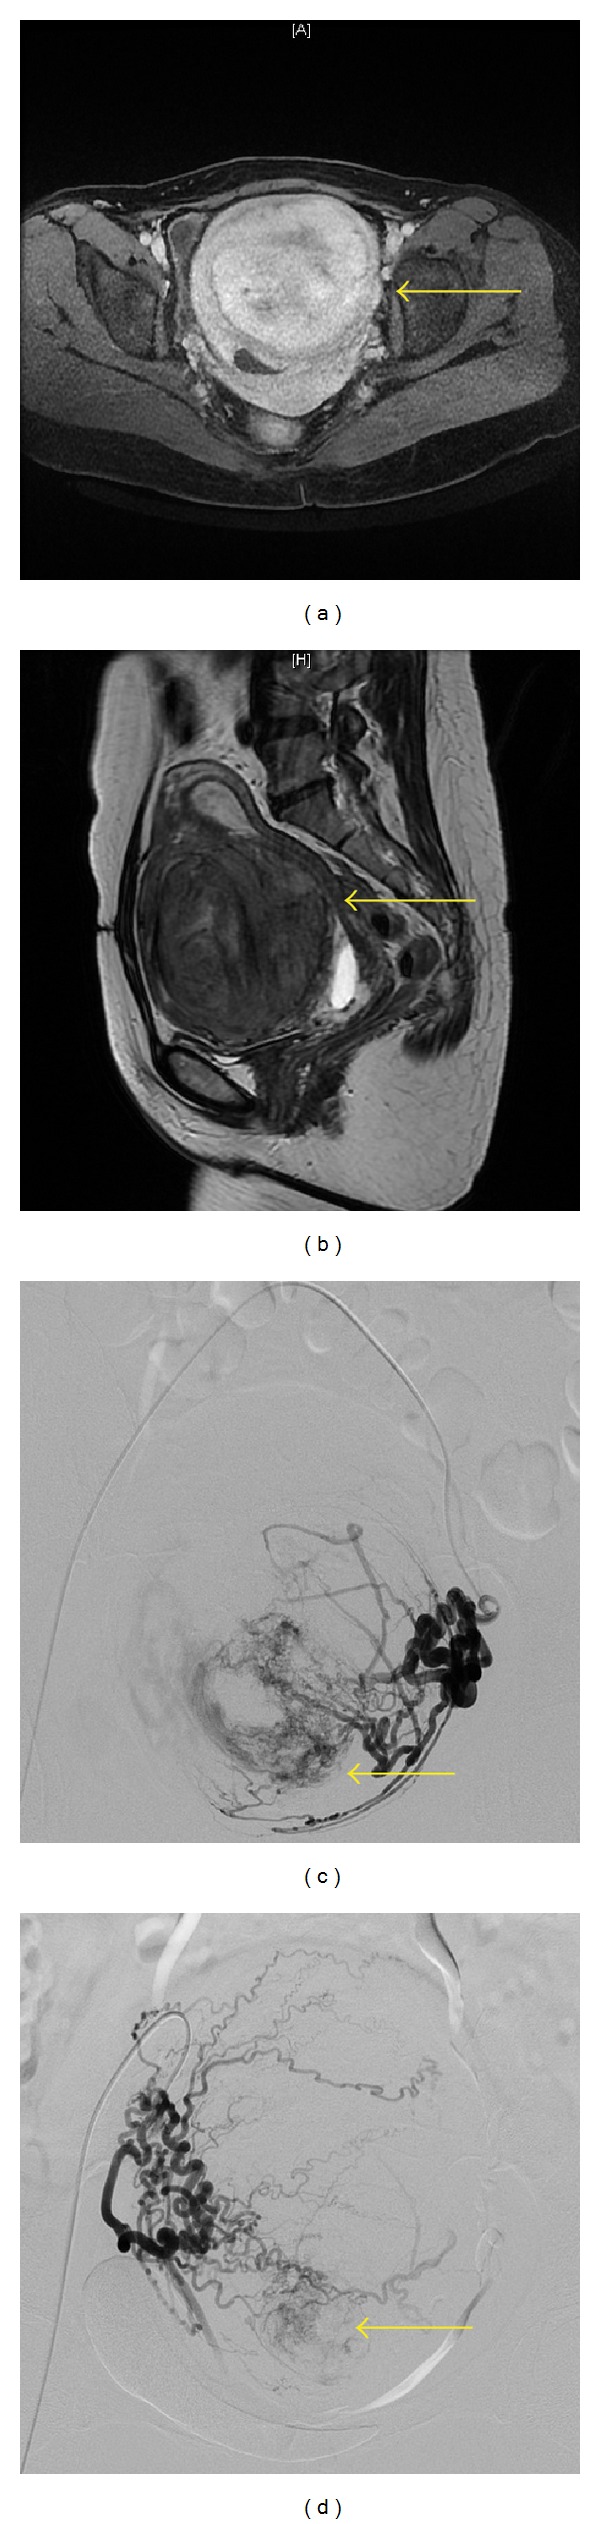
(a) Obvious and homogenous enhancement of the hysteromyoma and gestational sac after Gd-DTPA administration on axial T1-weighted imaging (arrow). (b) Giant hysteromyoma (9.9 cm × 9.5 cm × 9.8 cm) showing almost equal signal intensity on sagittal T2-weighted imaging (arrow). (c, d) Selective bilateral uterine arteries DSA showing persistence of the prominently enlarged and tangled vessels in the uterine and gestational sac measuring about 2.5 cm × 2 cm (arrow).
